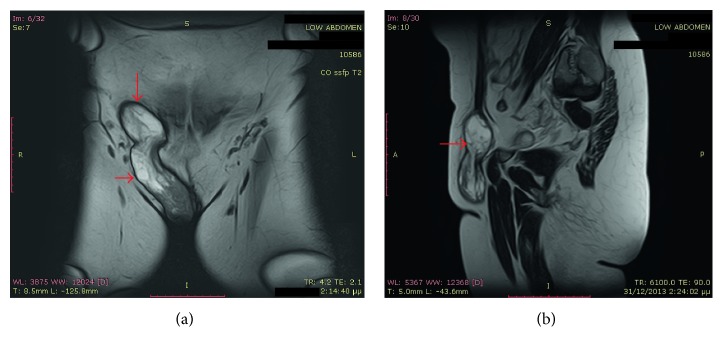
MRI T2W coronal and sagittal images showing a mass of the right round ligament of the uterus (red arrows).
